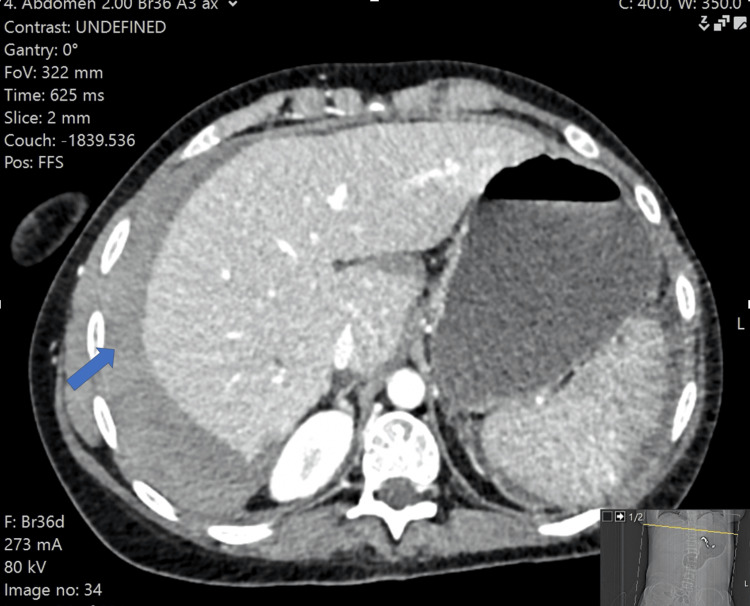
CT imaging of a case of haemorrhagic gallbladder. Haemoperitoneum with large perihepatic collection (arrow).
